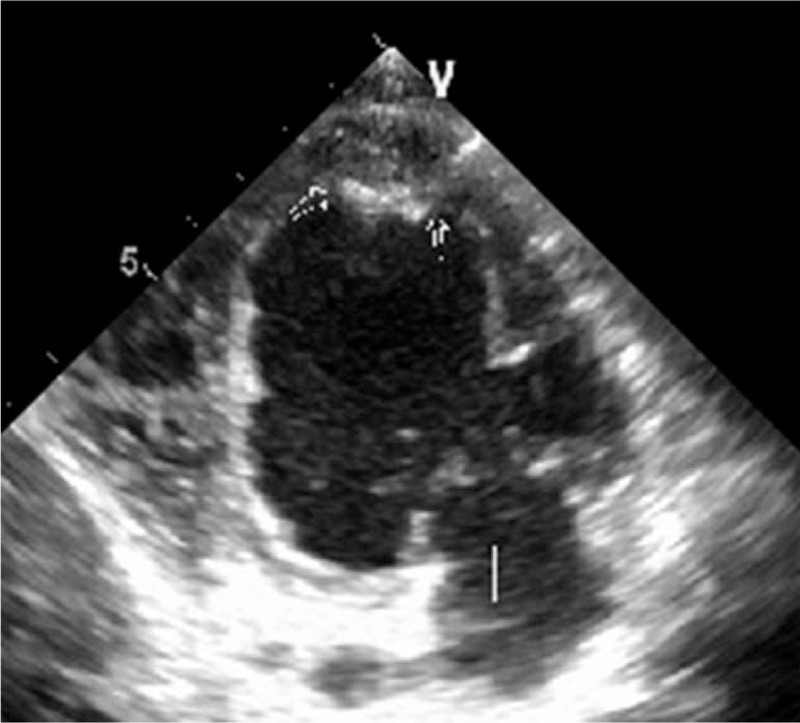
Two-dimensional echocardiography in the apical 4 chamber view showing a mass compatible with a large thrombus on the endocardial surface of the apex of the left ventricle.
